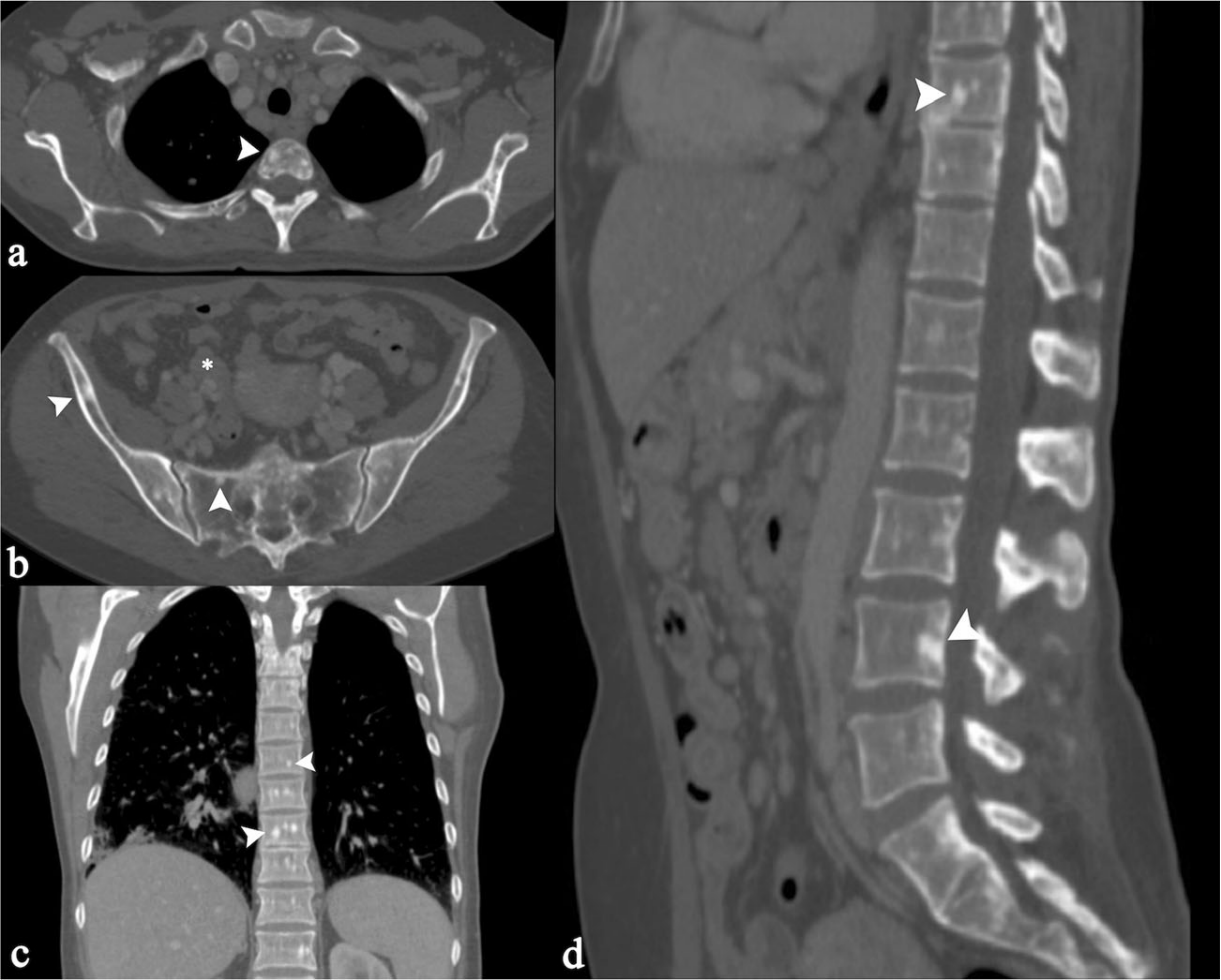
49 year-old woman with axial skeleton involvement. Axial CT images show osteosclerotic lesions (*arrowheads*) of dorsal vertebrae (Fig. 18a), sacrum (Fig. 18b), and bilateral iliac crest (Fig. 18b). A lymphadenopathy can be also observed on Fig. 18b (*white asterisk*). Coronal reconstruction clearly depicts small osteosclerotic foci in dorsal vertebrae. Sagittal reconstruction (Fig. 18d) demonstrates lesions in contiguous or non-contiguous vertebrae in the dorsal and lumbar spine
